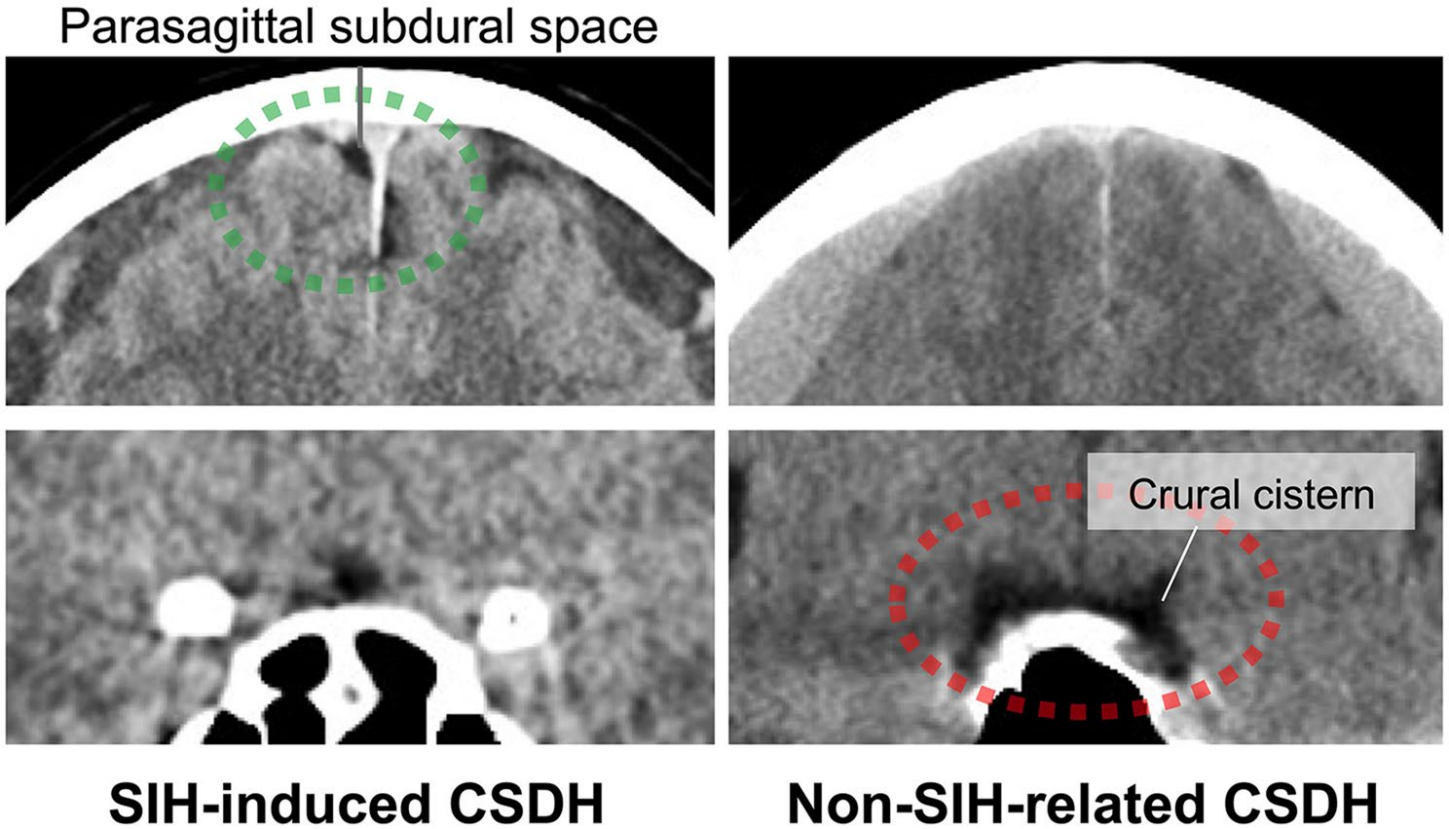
Representative coronal CT image of bilateral CSDH cases of SIH-induced and non-SIH-related
